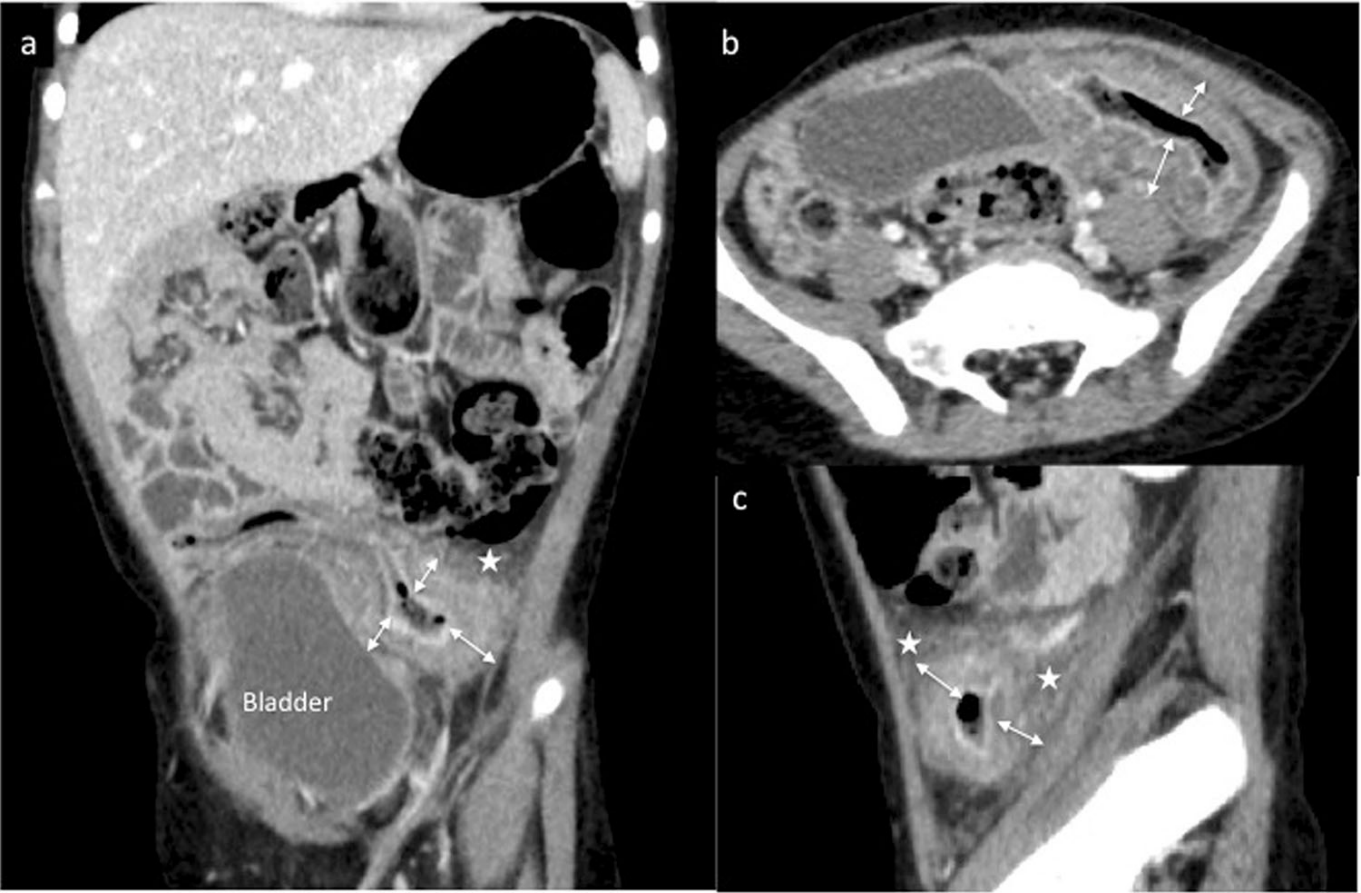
Abdominal CT findings. a Coronal view, b transverse view, c sagittal view. Contrast-enhanced abdominal CT shows thickening of the bowel wall localized to the sigmoid colon (*double-headed arrows*). Inflammation of fat around the sigmoid colon is also apparent (*stars*). The remaining parts of the gastrointestinal tract are almost normal
